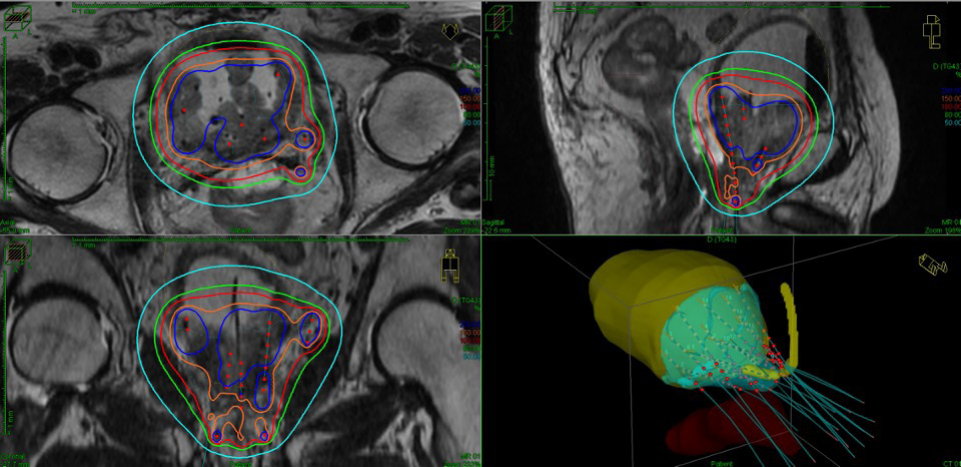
MRI image fused with planning CT after interstitial needle implantation.
